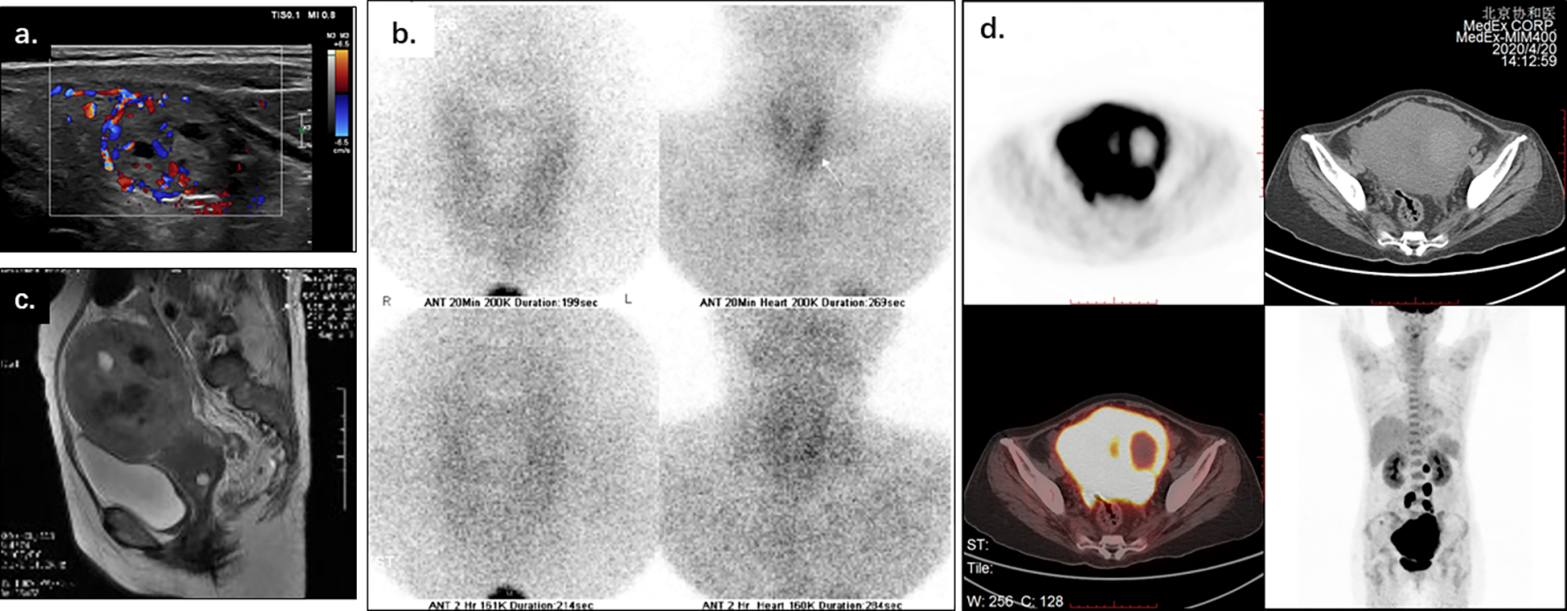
Thyroid ultrasonography, 99m Tc-MIBG, pelvic MRI, and PET-CT of a 56-year-old woman with life-threatening hypercalcemia. Thyroid ultrasonography (A) and 99m Tc-MIBG (B) showed a nodule inferior the left thyroid lobe, which may origin from parathyroid. Pelvic MRI (C) showed the uterus enlarged, with disrupted junctional zone, thickened myometrium, and abnormal signals in the uterine cavity. PET-CT (D) showed the irregularly enlarged uterus with increased SUV value (max to 15.7), as while multiple enlarged lymph nodes along the uterus, abdominal aorta, and bilateral common iliac arteries (SUVmax 15.5).
